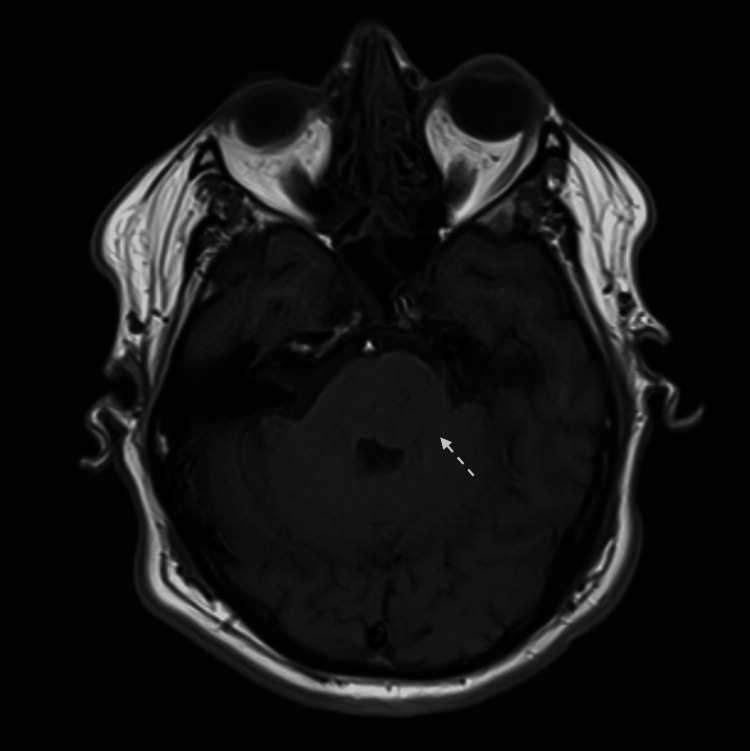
MRI brain T1 view showing asymmetry between right and left pons.
